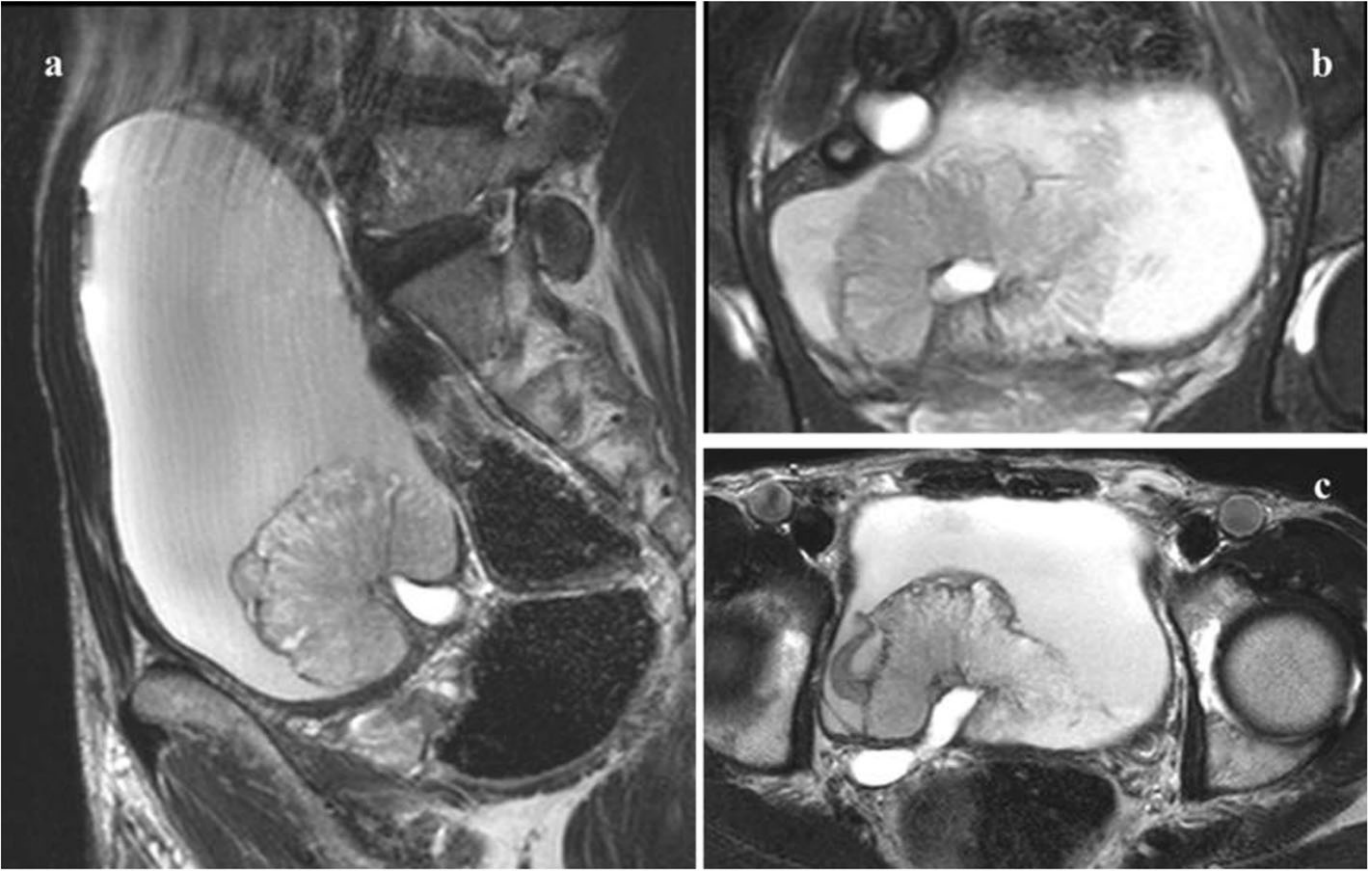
T2WI images of bladder tumors located around the ureter orifice on all scanning planes. a T2-weighted imaging of bladder tumor around the ureter orifice on the sagittal plane; b T2-weighted imaging on the coronal plane; c T2-weighted imaging on the transverse plane
